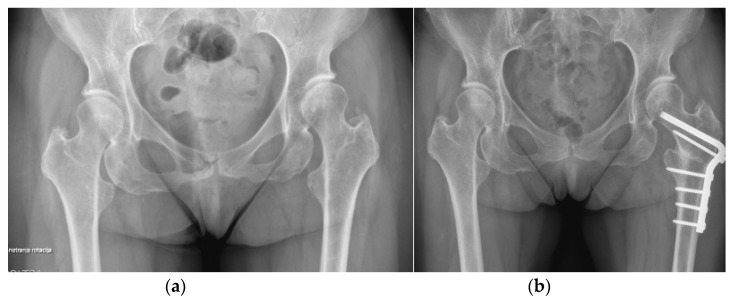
Varus femoral osteotomy. (a) preoperative X-ray; (b) postoperative X-ray.
